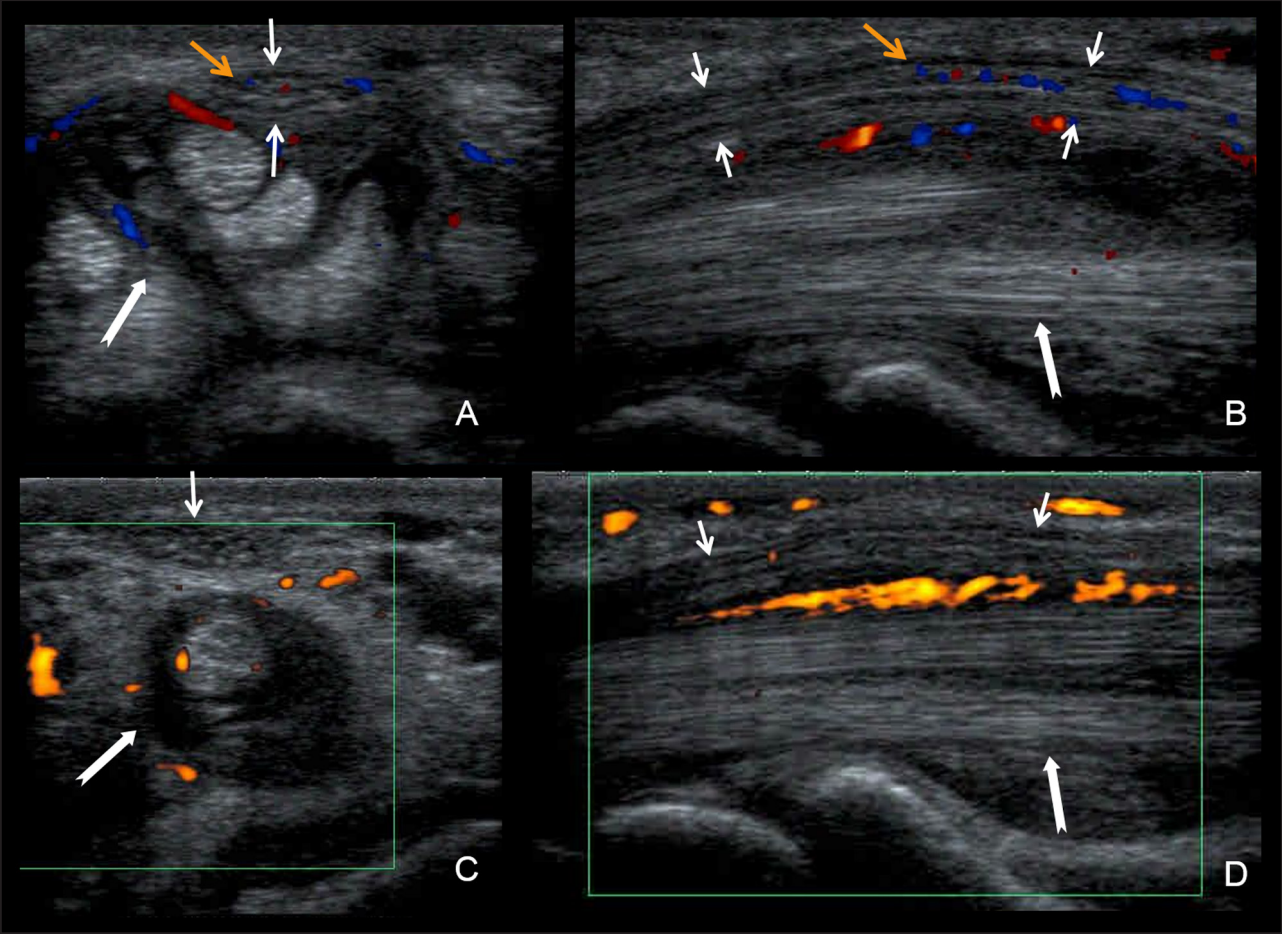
Flexor tenosynovitis. Axial (A, C) and sagittal (B, D) views on US Doppler examination. See the effusion and the hyperemia (white arrows) in the tendon sheath and the intraneural hyperemia (orange arrows) in the median nerve.
